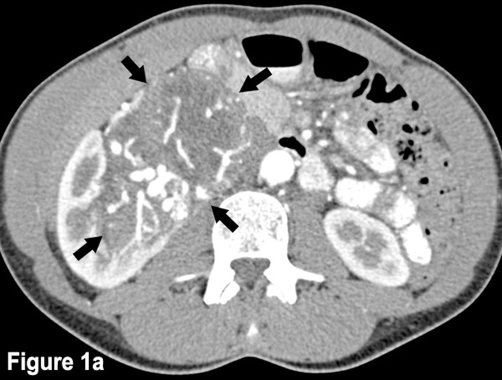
Contrast-enhanced CT scan of the abdomen shows a well-defined, highly vascularized renal tumor. The mass extends towards the renal pelvis and the right kidney is laterally displaced. Tumor borders are indicated by arrows.
